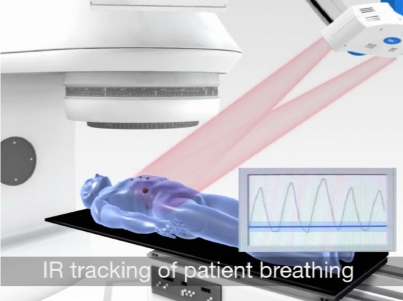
Video clip on principle of the BrainLab Exac-Tract X-ray treatment (courtesy of BrainLab, Germany) (available for download from http://www.biij.org/2006/1/e19).
